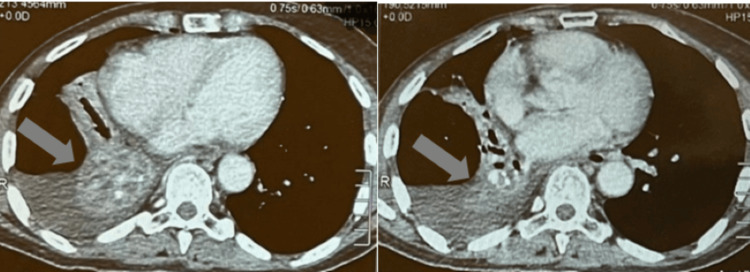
Chest CT scan showing a right lower lobe atelectasis.
